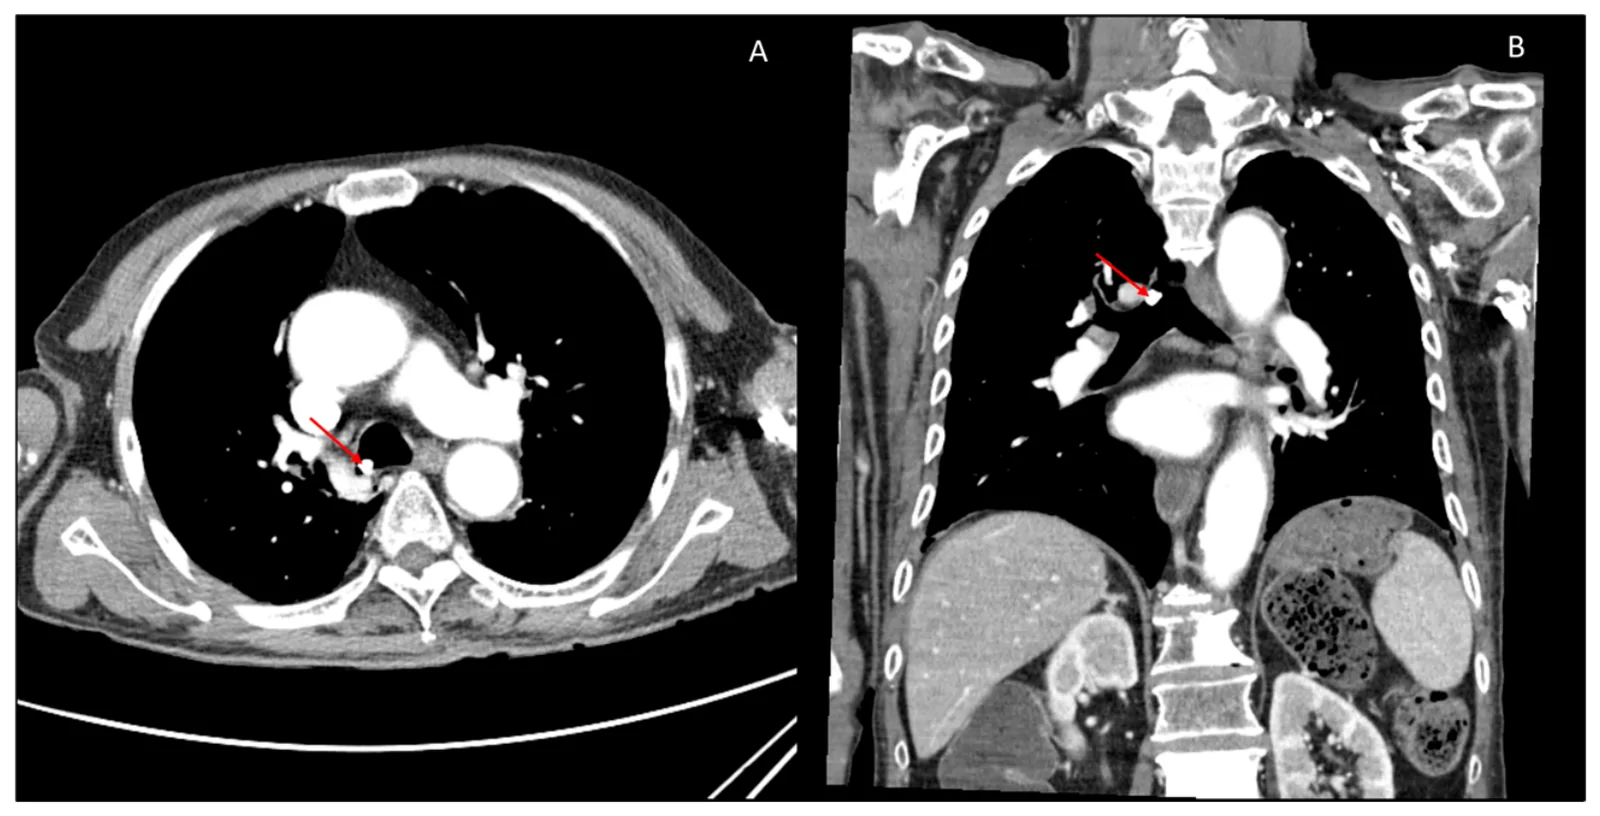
Initial contrast-enhanced chest computed tomography. (A) Axial CT image demonstrating a tooth-like radiopaque foreign body in the right main bronchus (red arrow). (B) Coronal reconstructed CT image demonstrating the same foreign body in the right main bronchus (red arrow).
